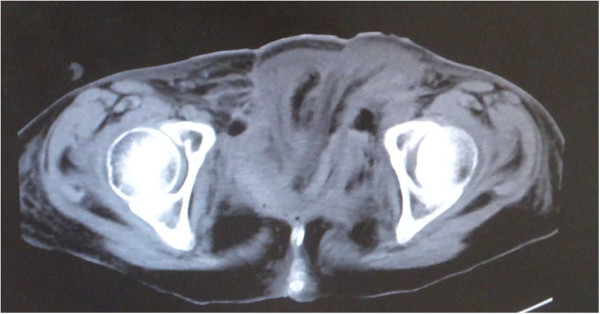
Computed tomography scan after 2 weeks showing the pelvic cavity filled with the transported muscle.
